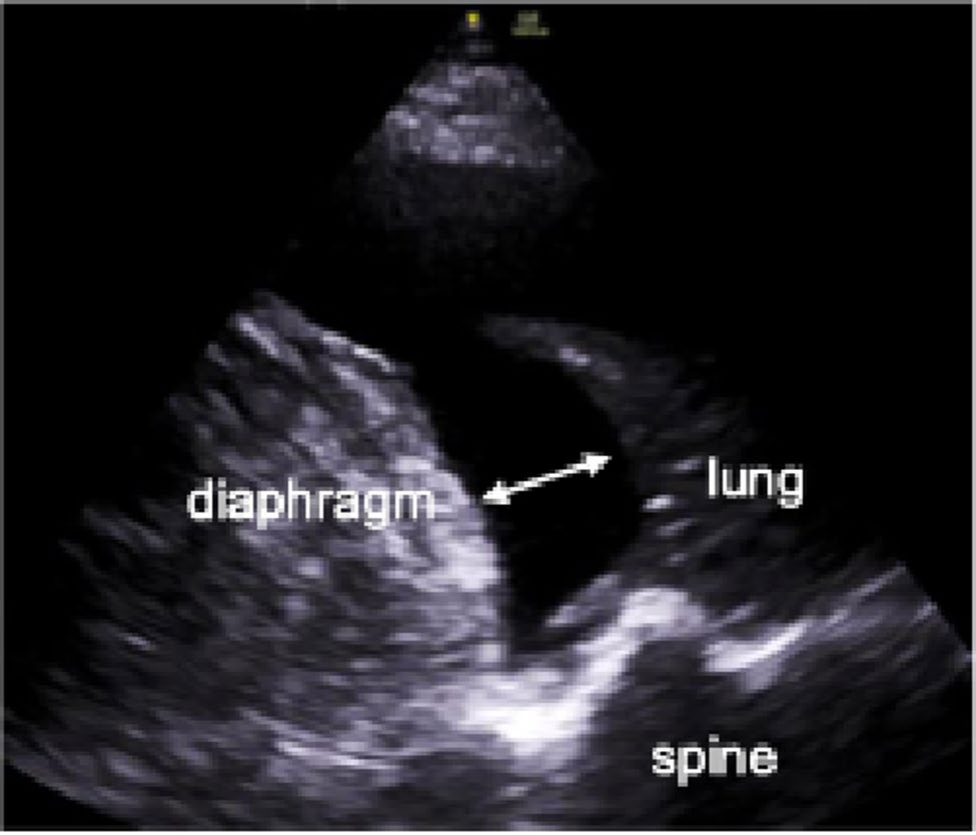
Ultrasound image of pleural effusion. Double arrow indicates largest distance between lung atelectasis and basal diaphragm
